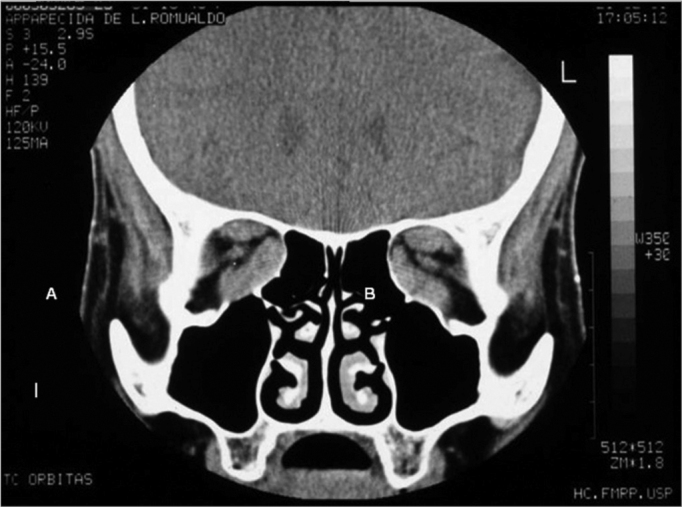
CT scans, coronal views (B) of a patient with Graves’ ophthalmopathy, showing the hypertrophy of the extrinsic ocular muscles.
